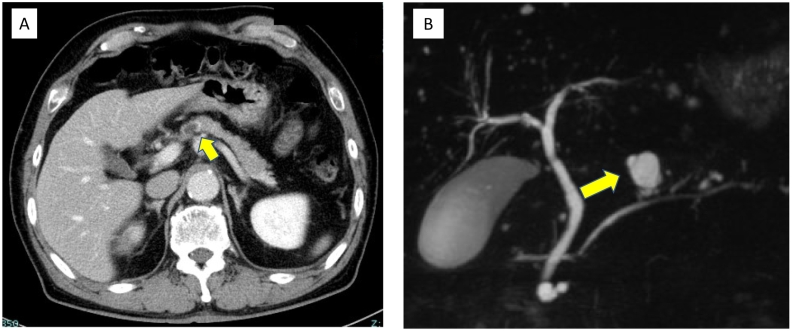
Imaging studies during the preoperative examination for esophagogastric junction cancer. A: Contrast-enhanced computed tomography. A cystic lesion is observed in the pancreatic body. B: Magnetic resonance cholangiopancreatography: cystic lesion connected to the main pancreatic duct is recognized in the pancreatic body.
